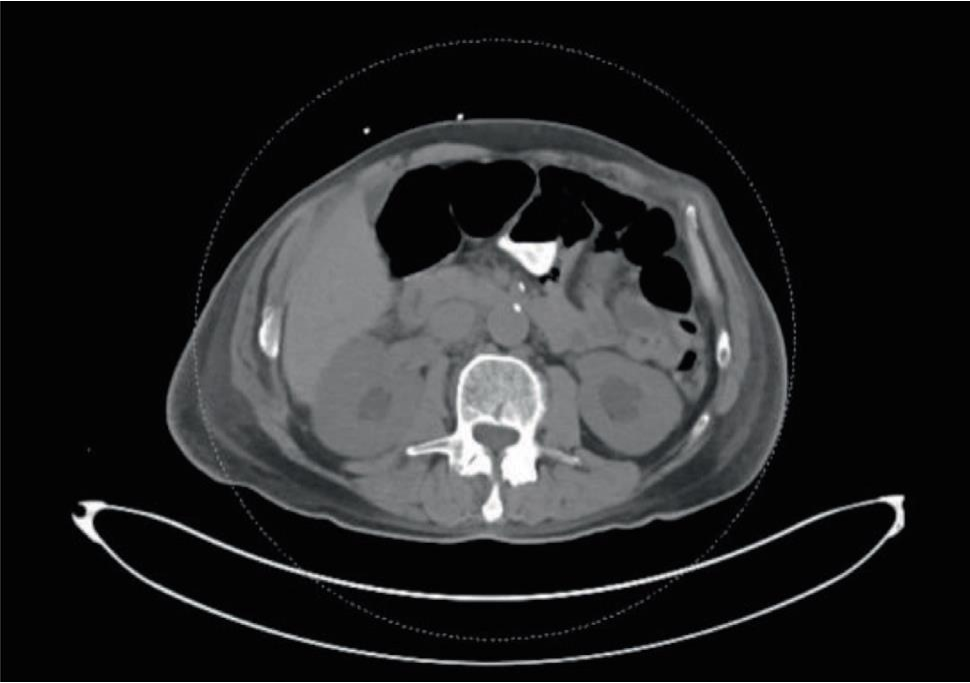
CT scan on admission showing bilateral hydronephrosis.
